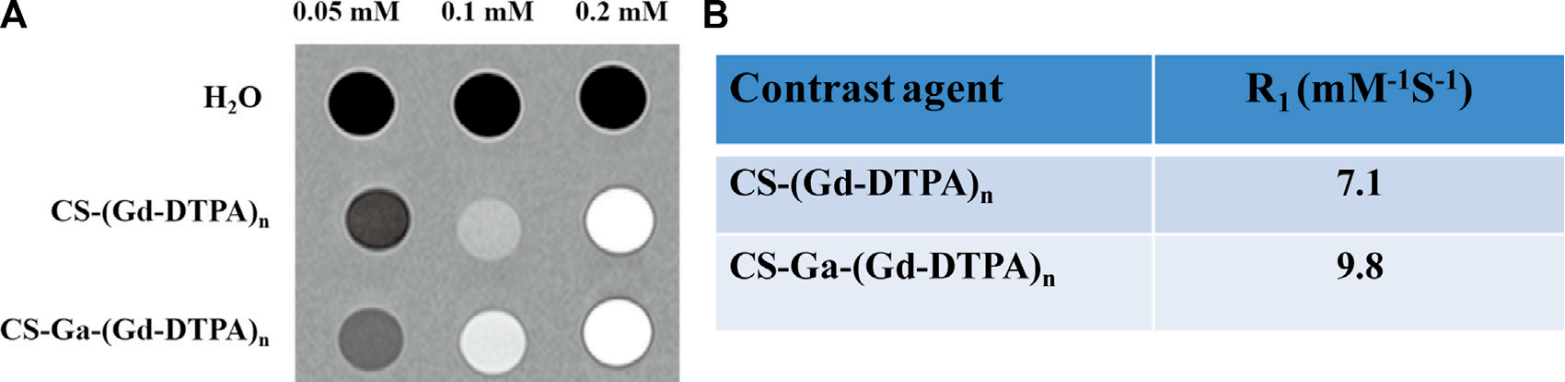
(A) T_1_-weighted MR images of H_2_O, CS-(Gd-DTPA)_n_ and CS-Ga-(Gd-DTPA)_n_. (B) The longitudinal relaxivity (R_1_) of CS-(Gd-DTPA)_n_ and CS-Ga-(Gd-DTPA)_n_.
